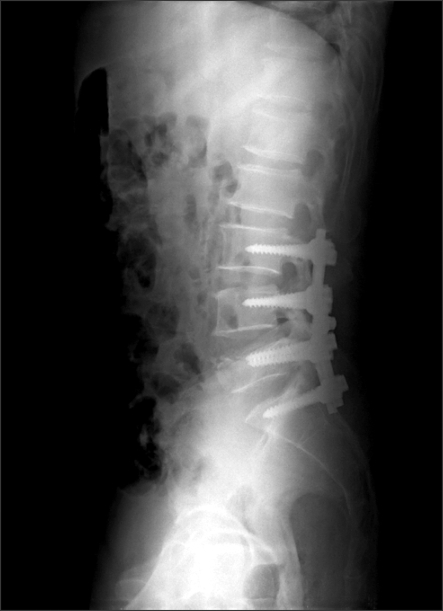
Postoperative radiographs showed posterior segmental fixation from L3 to S1.
